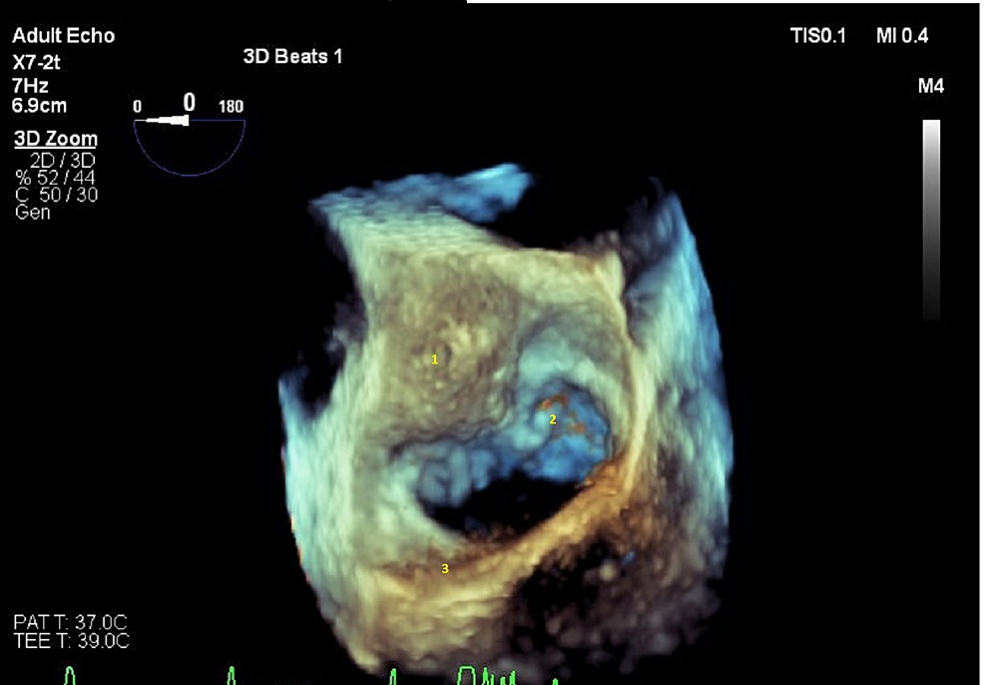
Three-dimensional transesophageal echocardiogram of left atrial En-face view of the mitral valve showing: 1. Bulging of aortic root abscess encroaching left atrium. 2. Anterior leaflet of the mitral valve. 3. Posterior leaflet of the mitral valve.
